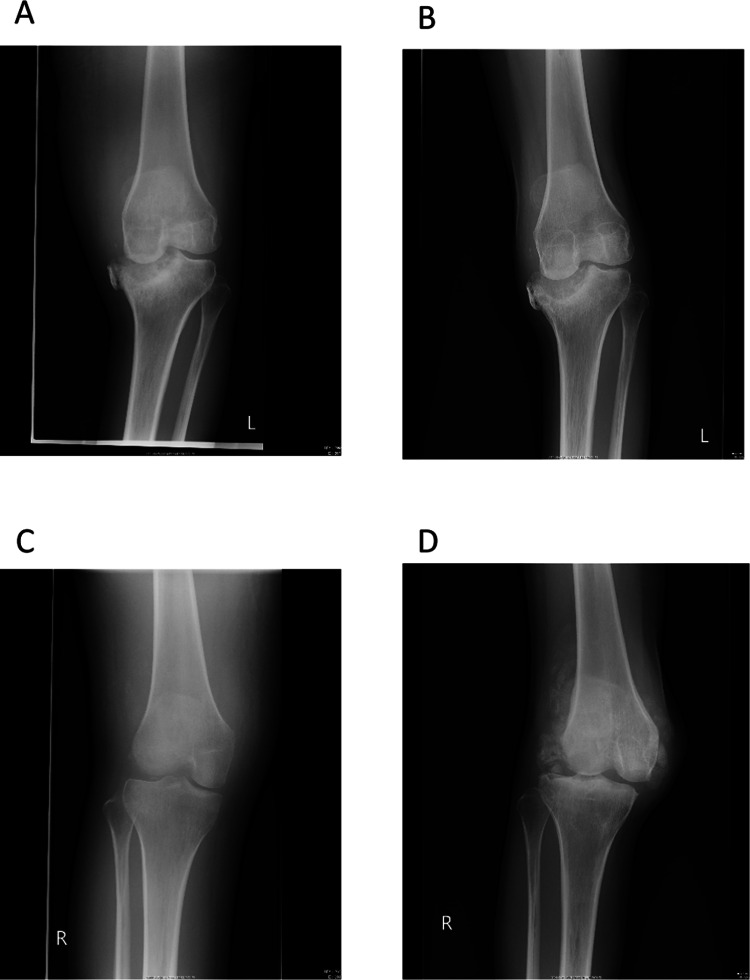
X-rays of the left (A, B) and right knee (C, D) at the time of hospitalization and one year later
